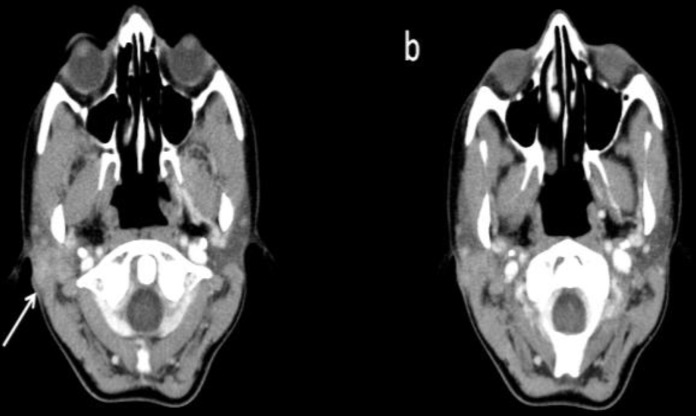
Contrast-enhanced neck CT scan: *a *is cephalad to *b* and depict a moderately enhancing mass in the posterior portion of the right parotid gland with a lobulated and partially indistinct margin. There is involvement of subcutaneous tissue (arrow
